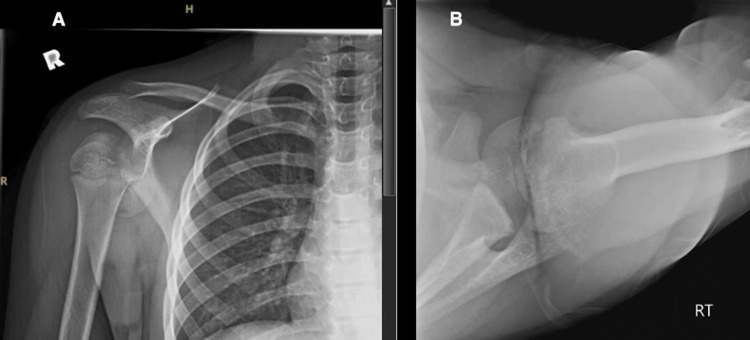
(A) Anteroposterior (AP) and (B) Velpeau radiographs showing an SH III fracture-dislocation of the right shoulder.
